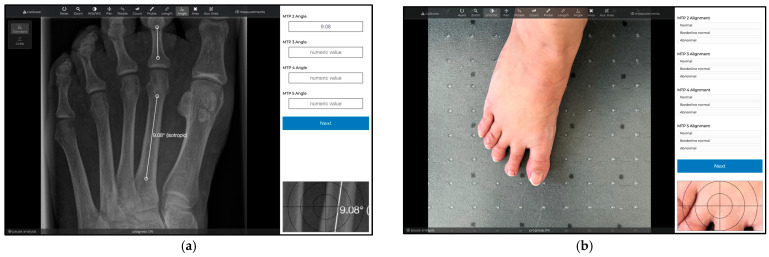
Web-based online analysis tool Tyche™. (a) Assessment of clinical images, (b) assessment of radiographic images.
